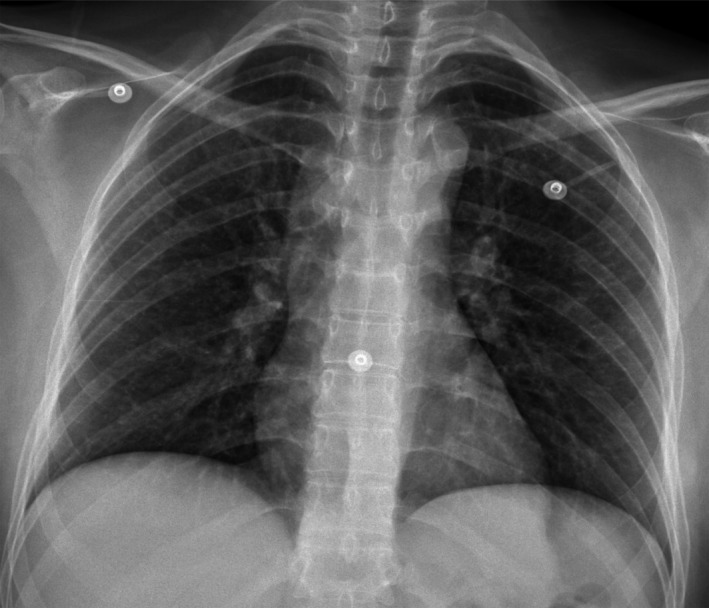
Chest radiography demonstrating the presence of a diffuse reticulonodular interstitial pulmonary pattern and a discrete mediastinal widening.
